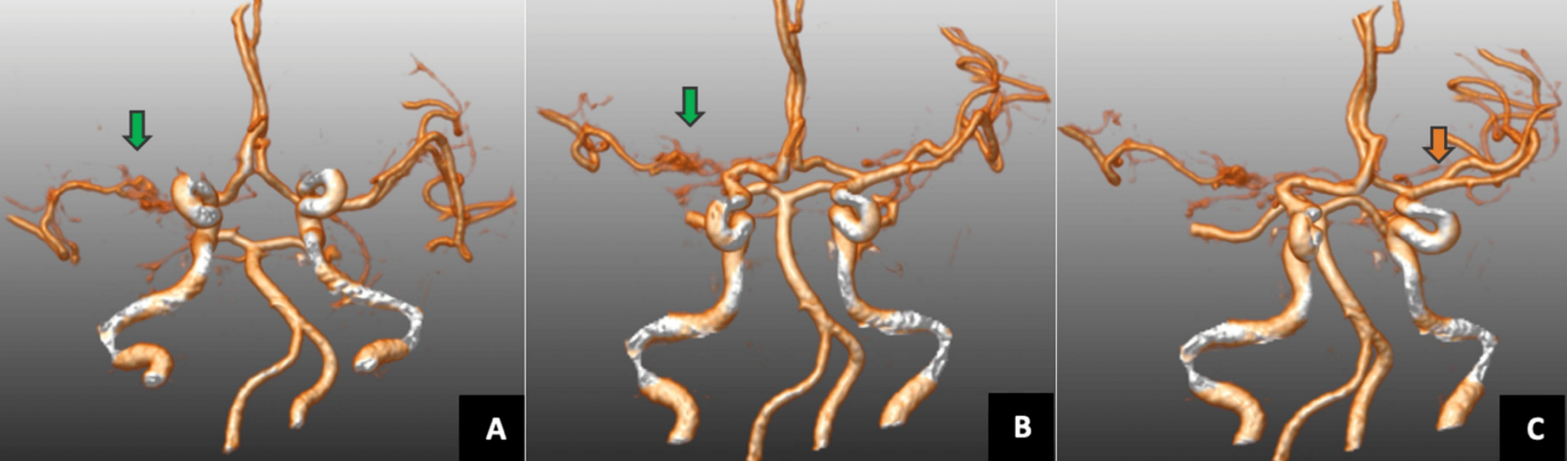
CT brain 3D reconstruction It showed an abnormal plexiform network in the right M1 segment (A and B, green arrow) and early division of the left middle cerebral artery with trifurcation (C, orange arrow).
